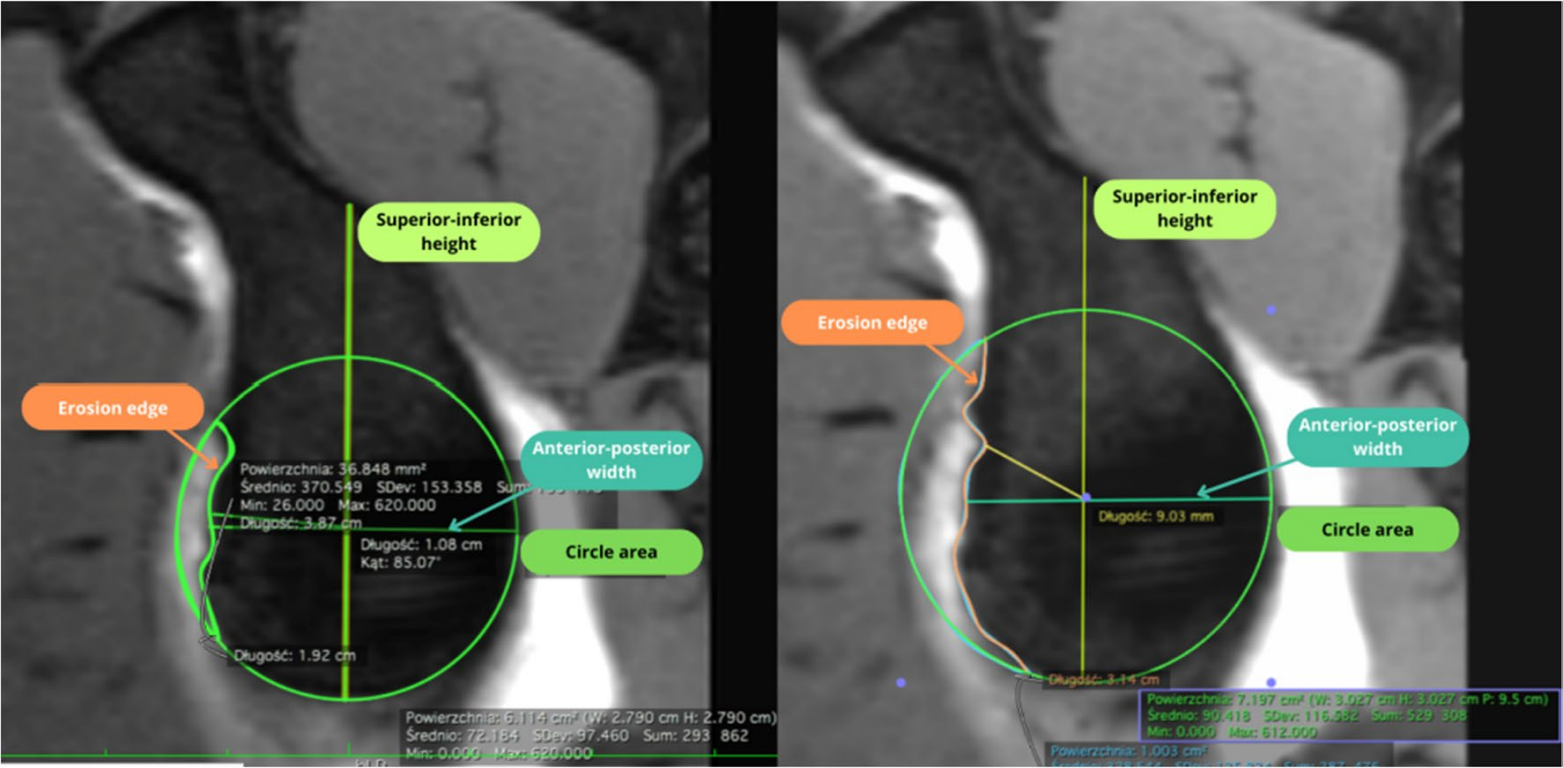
Example of difference in measurements between two investigators on the same MRI scan
